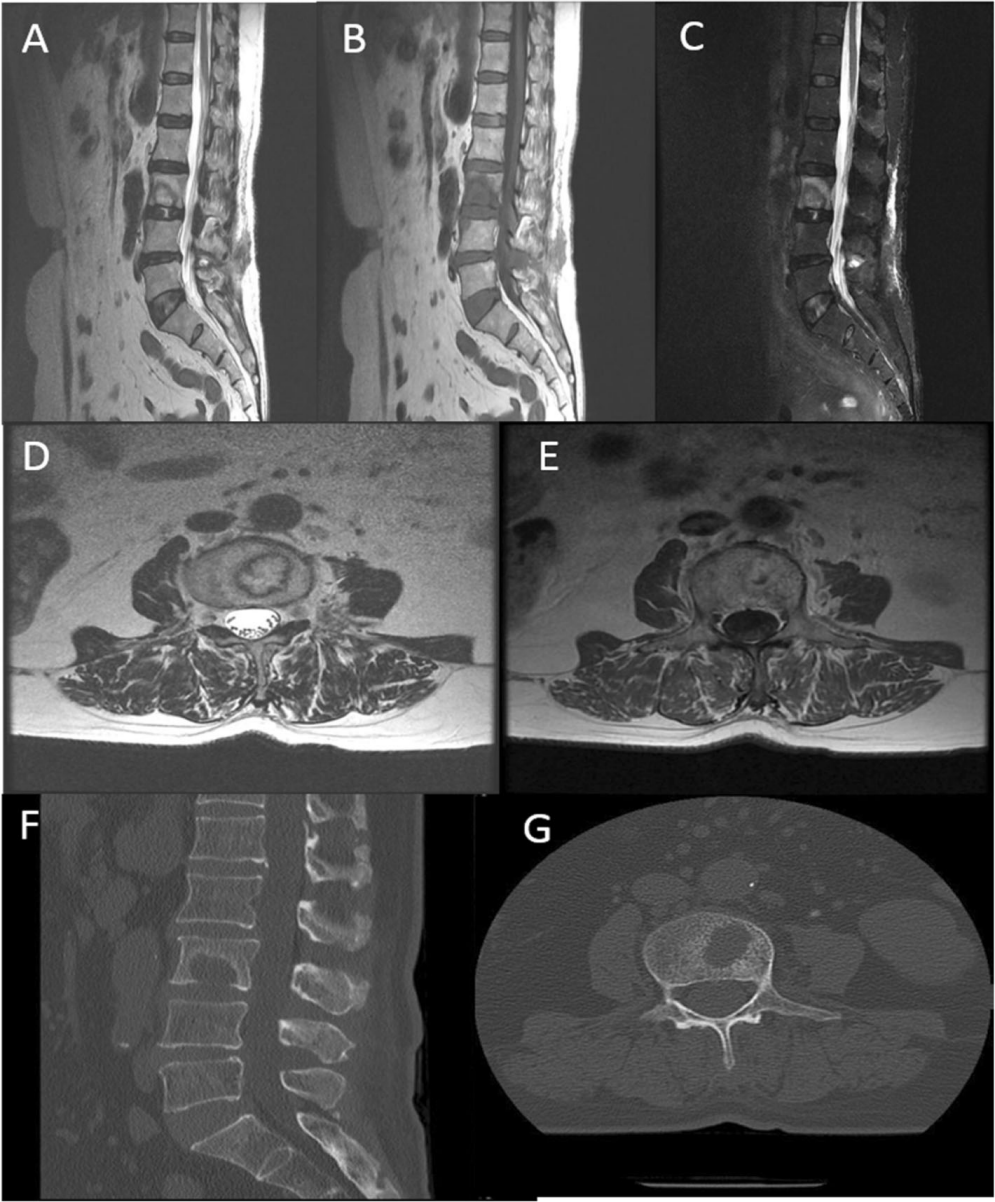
Repeat MRI (a, b) T1 weighted sagittal image and (c) STIR image showing increased signal intensity in L3 vertebra with anterio-superior part of L4. b Follow-up T2 weighted images showing decreased signal intensity suggestive of extensive marrow oedema. Repeat CT scan saggital (f) and axial (g) section showing increase in size of osteolytic lesion
